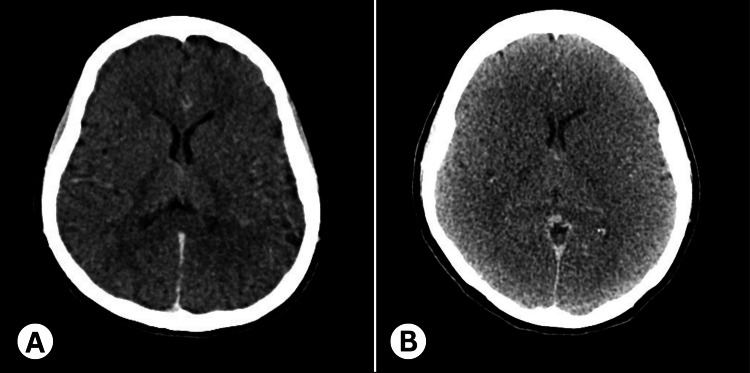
Computed tomography imaging of the brain (A) No evidence of hemorrhage or infarction (B) Global hypoxic-ischemic injury to the brain
